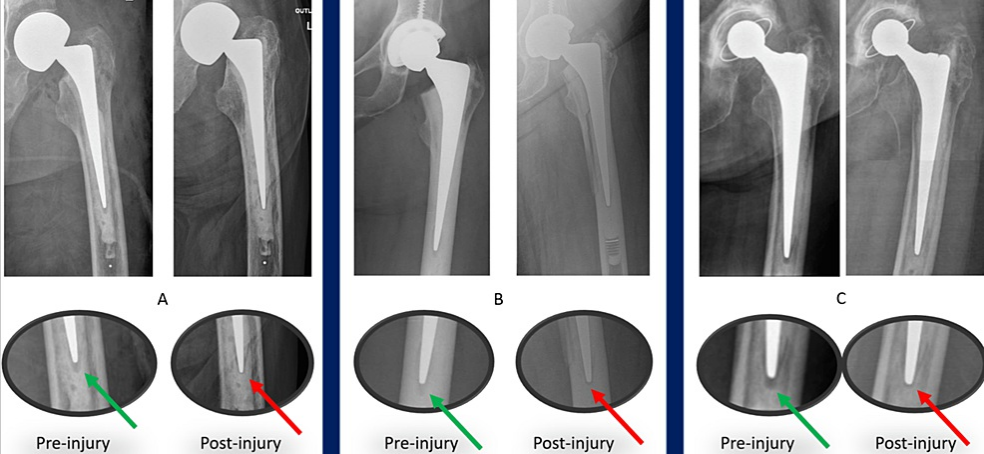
Complete obliteration AP radiographs of three separate patients (A-C) demonstrating pre-injury normal features after index arthroplasty and post-injury positive ‘SINC’ sign with complete obliteration of the lucency representing the centraliser.
